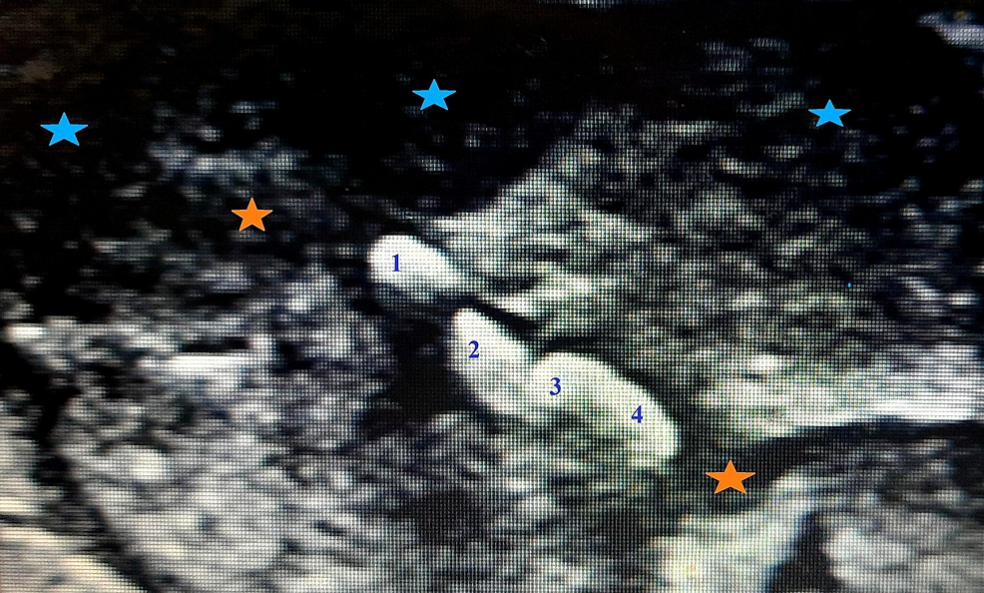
High-resolution ultrasonography image of the submandibular gland demonstrating steinstrasse in the Wharton’s duct causing mild dilatation consistent with features of submandibular sialolithiasis. Note the stacked calculi (numbers), dilated Wharton’s duct with sludge (orange stars), and hypoechoic areas (blue stars) within the submandibular gland consistent with features of sialadenitis.
